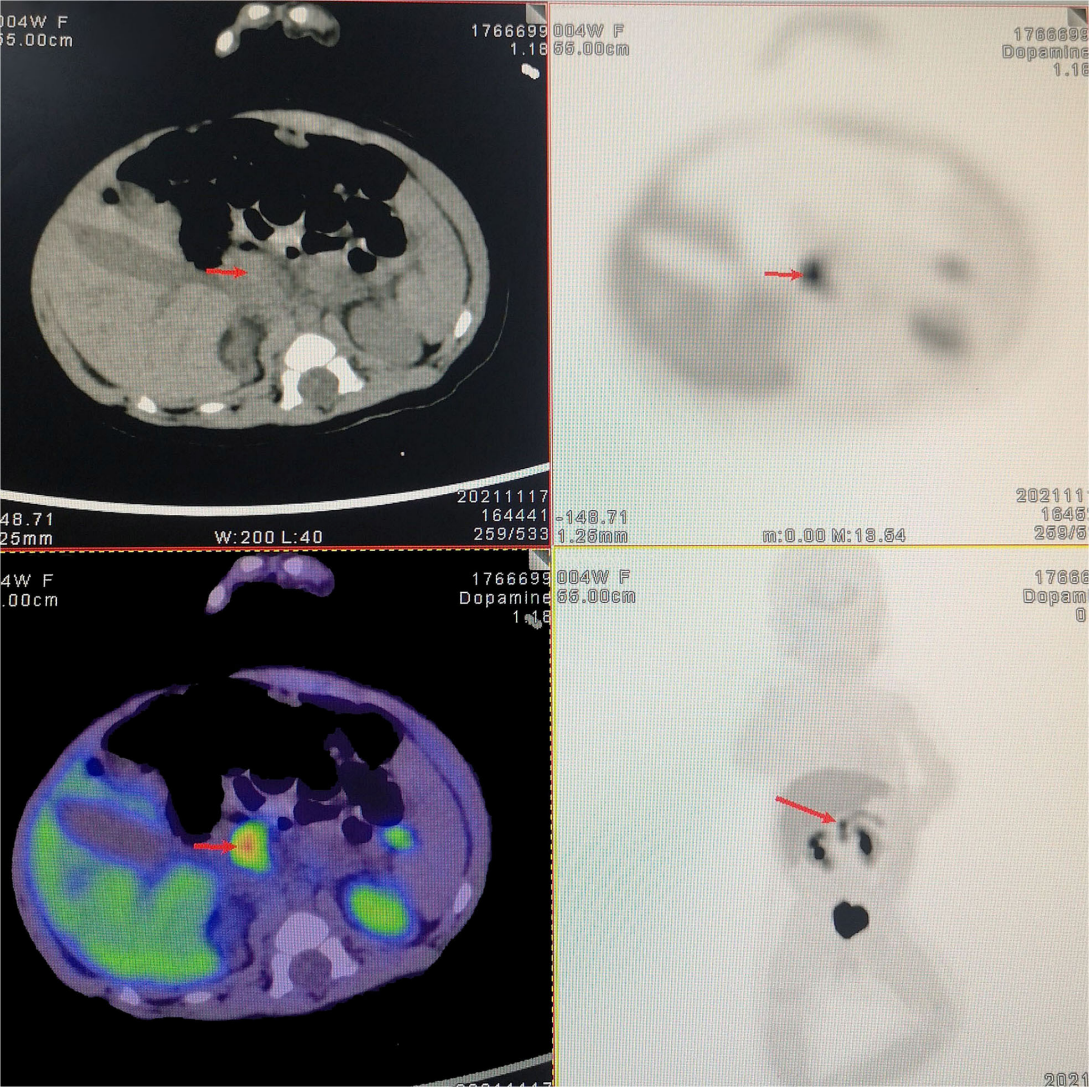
18-Fluoro-dihydroxyphenylalanine (18F-DOPA) positron emission tomography/computed tomography (PET/CT) scan. Preoperative 18F-DOPA PET/CT shows a focal lesion in the head of the pancreas.
